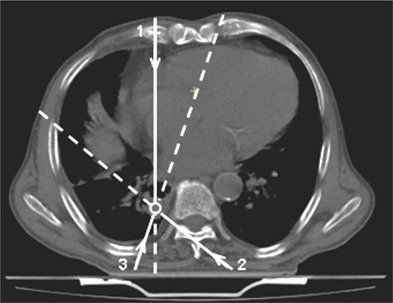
Isocenter CT scans with three beams at 0° (1), 130° (2) and 200° (3) gantry angles. The circle shows the isocenter position.
